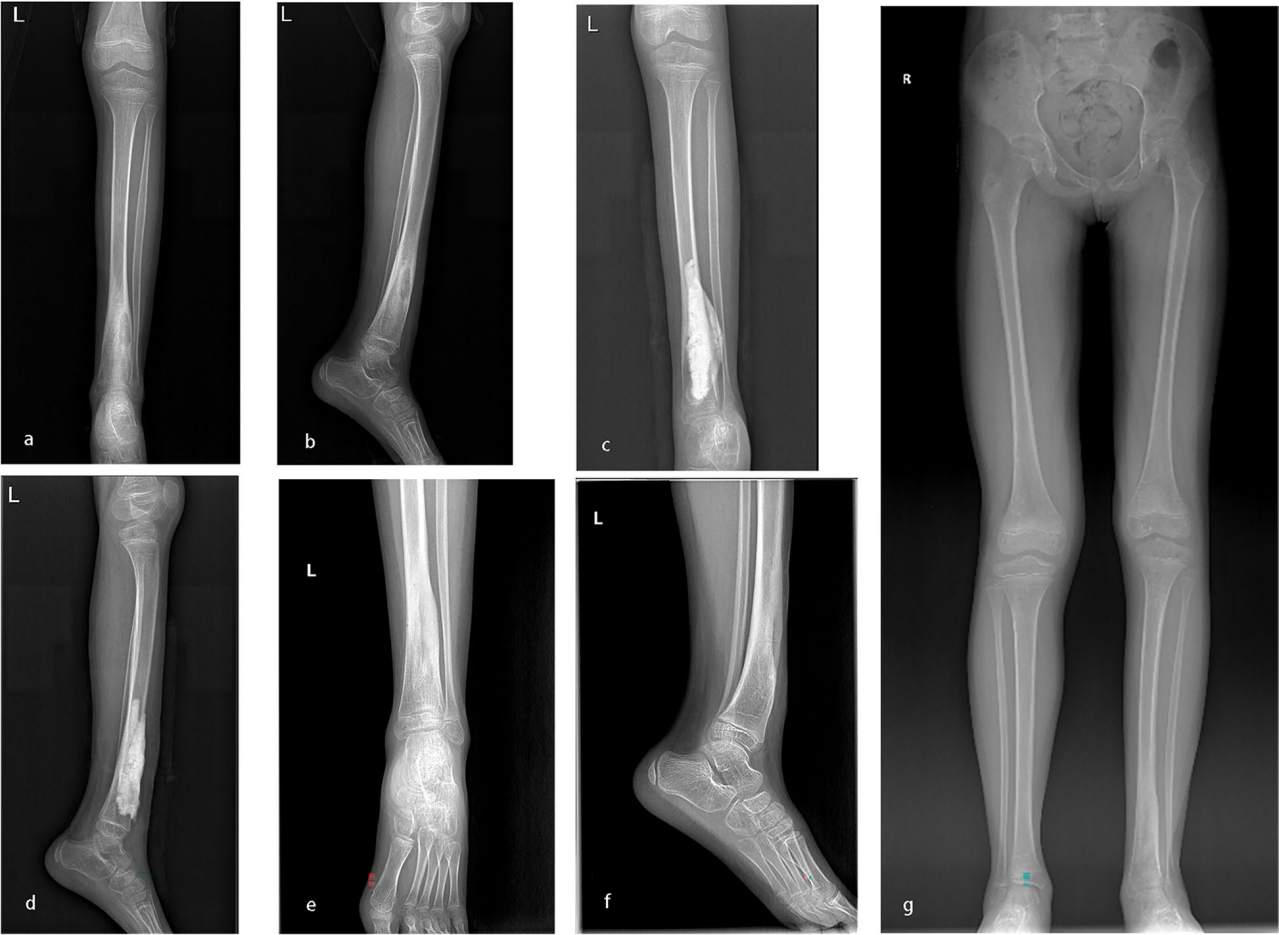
a-b: A seven-year-old girl suffered left distal tibial HO. c-d: Vancomycin impregnated CS was used to fill the void left by decompression and debridement. e–g: X-ray examination indicated acceleration of bone growth 12 months postoperatively
